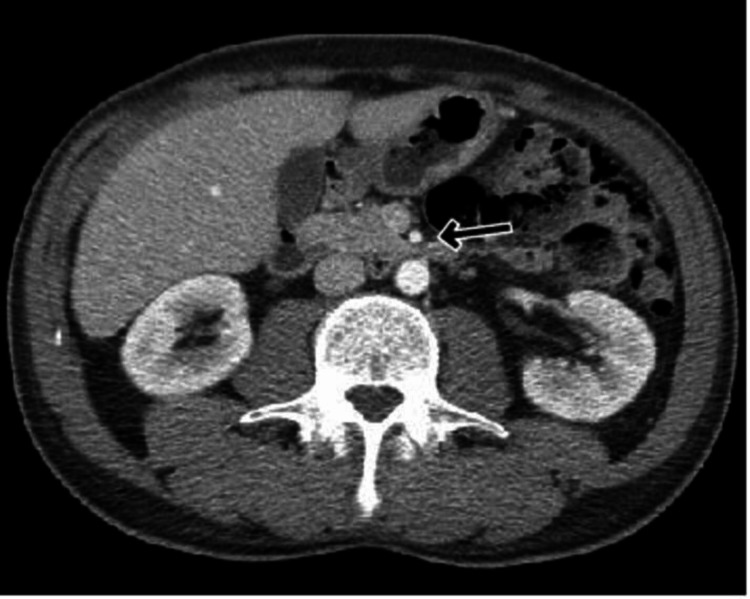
CT Abdomen Depicting an Aortomesenteric Distance of 6 mm Arrow pointing toward the distance between the aorta and superior mesenteric artery. The average normal aortomesenteric distance is 10-20 mm.
